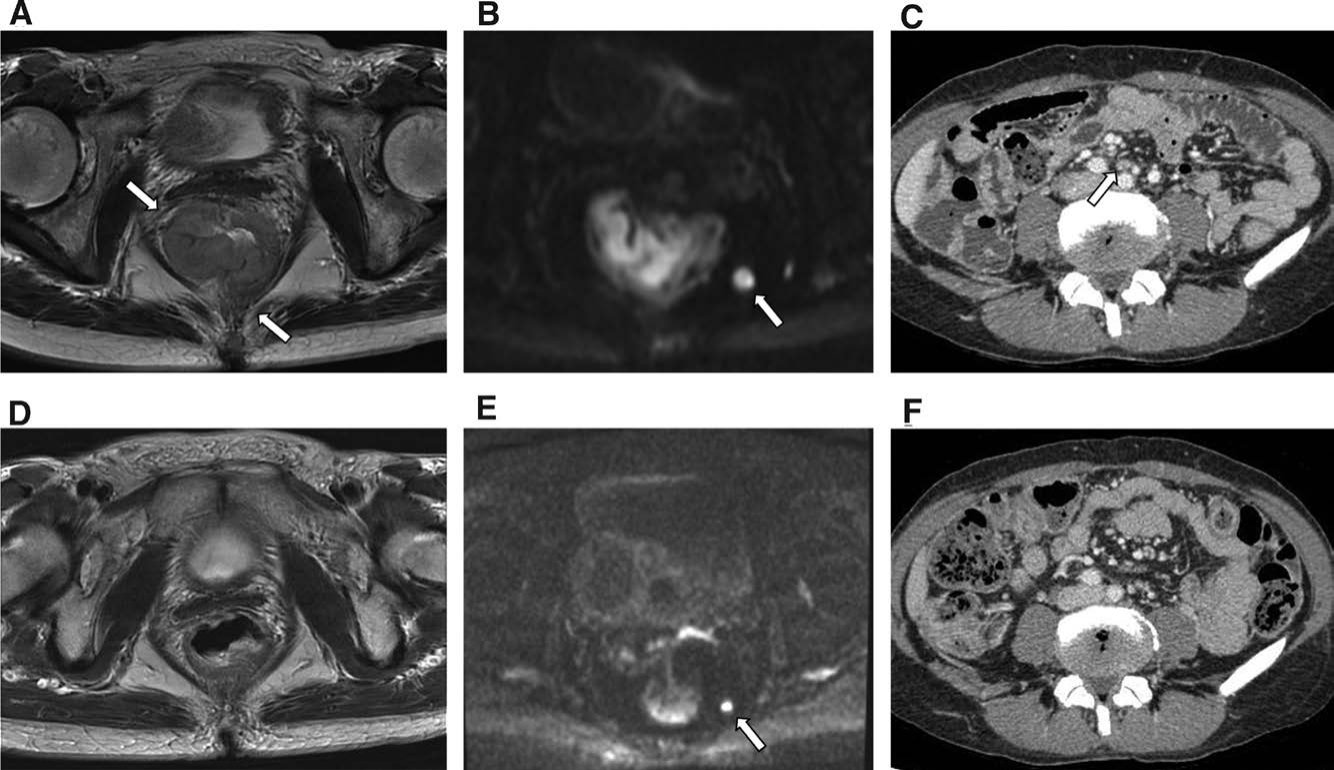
Findings of abdominal MRI and CT. (A) Abdominal contrast MRI shows the presence of a large tumor in the lower rectum. White arrows indicate the suspected extramural invasion. (B) Abdominal contrast MRI shows the presence of a round-shaped enlarged lymph node more than 10 mm in diameter at the pararectal region (white arrow). (C) Abdominal contrast CT indicated a lymph node 8 mm in diameter at the superior rectal arterial region (white arrow). (D) Abdominal contrast MRI indicating apparent tumor shrinkage. (E) Abdominal contrast MRI indicating apparent lymph node shrinkage (white arrow). (F) Abdominal contrast CT indicating that the previously seen enlarged lymph node has disappeared. CT = computerized tomography, MRI = magnetic resonance imaging.
